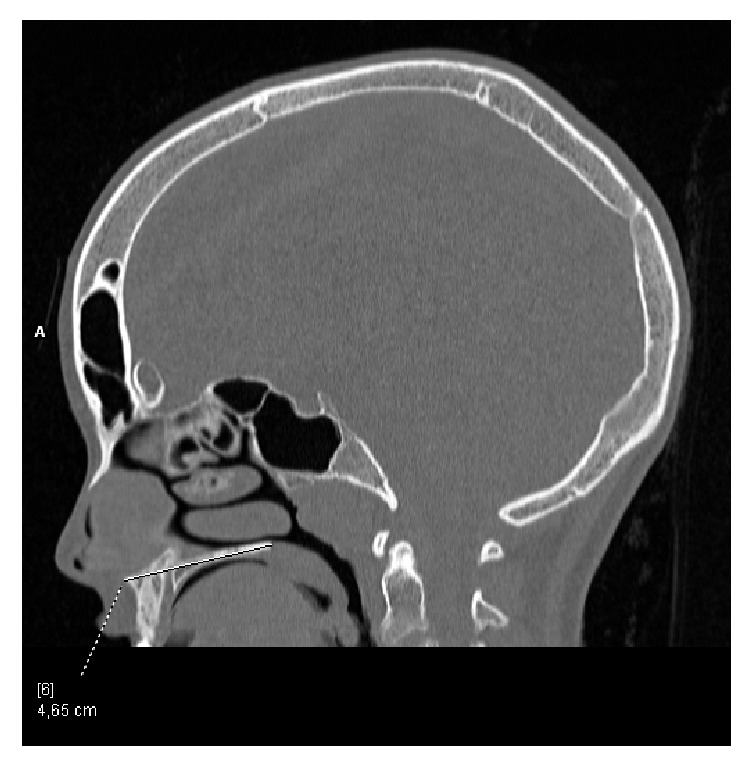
An example of ns-P distance marked on the CT image.
